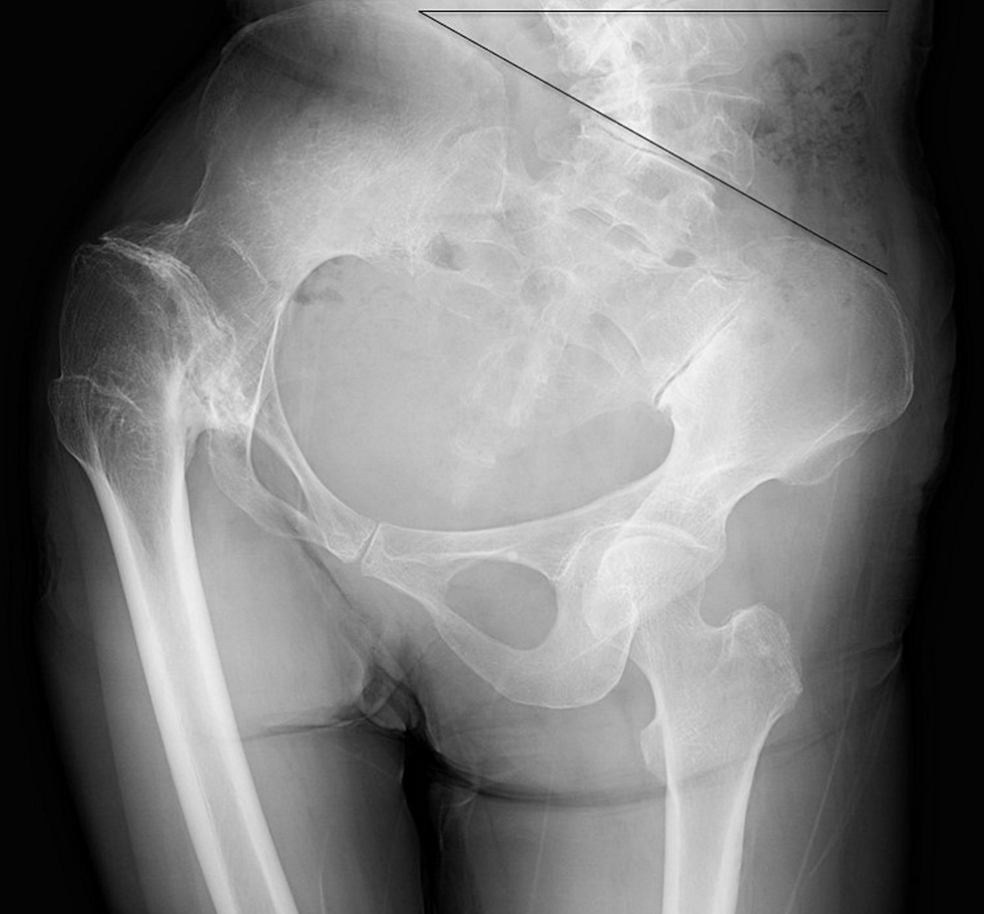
Preoperative antero-posterior radiographs of the hip joints. Preoperative antero-posterior radiographs show narrowing of the hip joint on the right side, caused by TB, with significant pelvic obliquity and adduction contracture of the hip.
